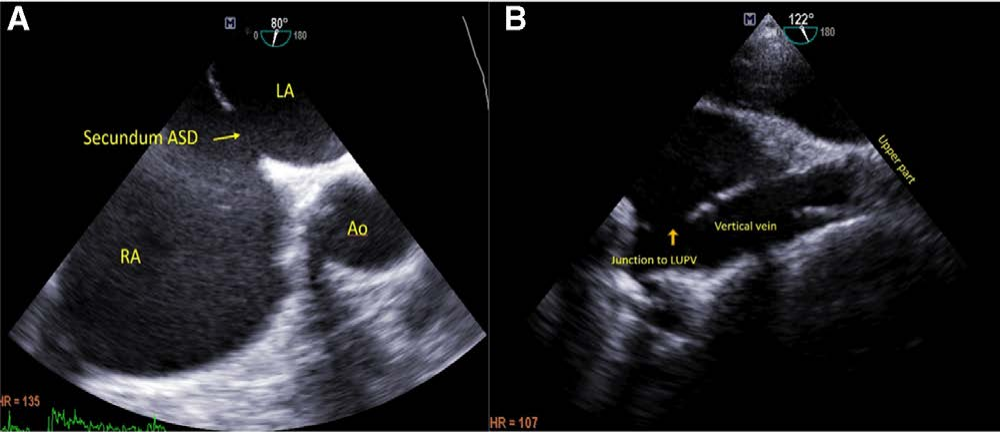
Transesophageal echocardiography images. (a) Two-dimensional TEE image shows atrial septal defect and (b) vertical vein before device closure. Ao = aorta, LA = left atrium, LUPV = left upper pulmonary vein, RA = right atrium, TEE = trans esophageal echocardiography.
